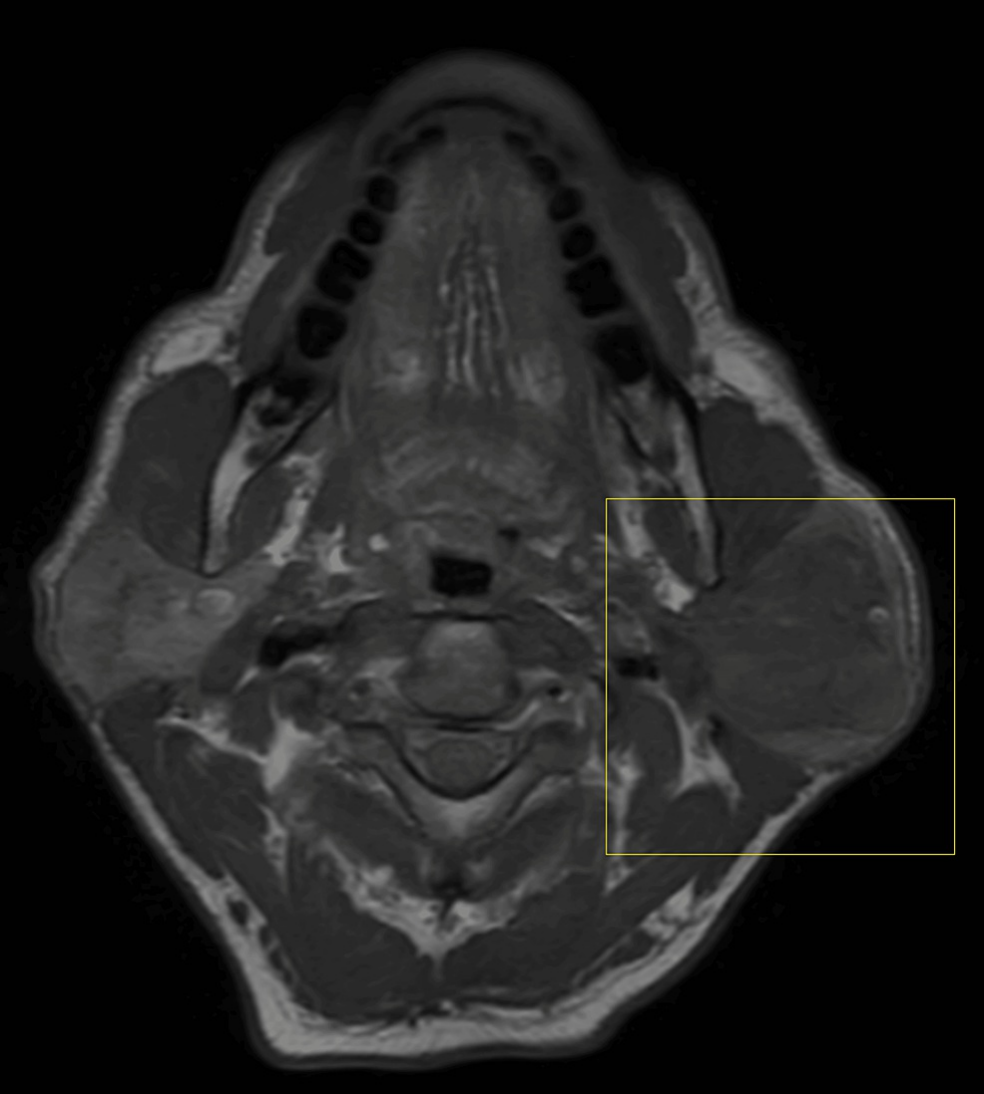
TIWI axial MRI image The highlighted area shows the physical presentation of parotid gland tumor T1WI, T1-weighted image
